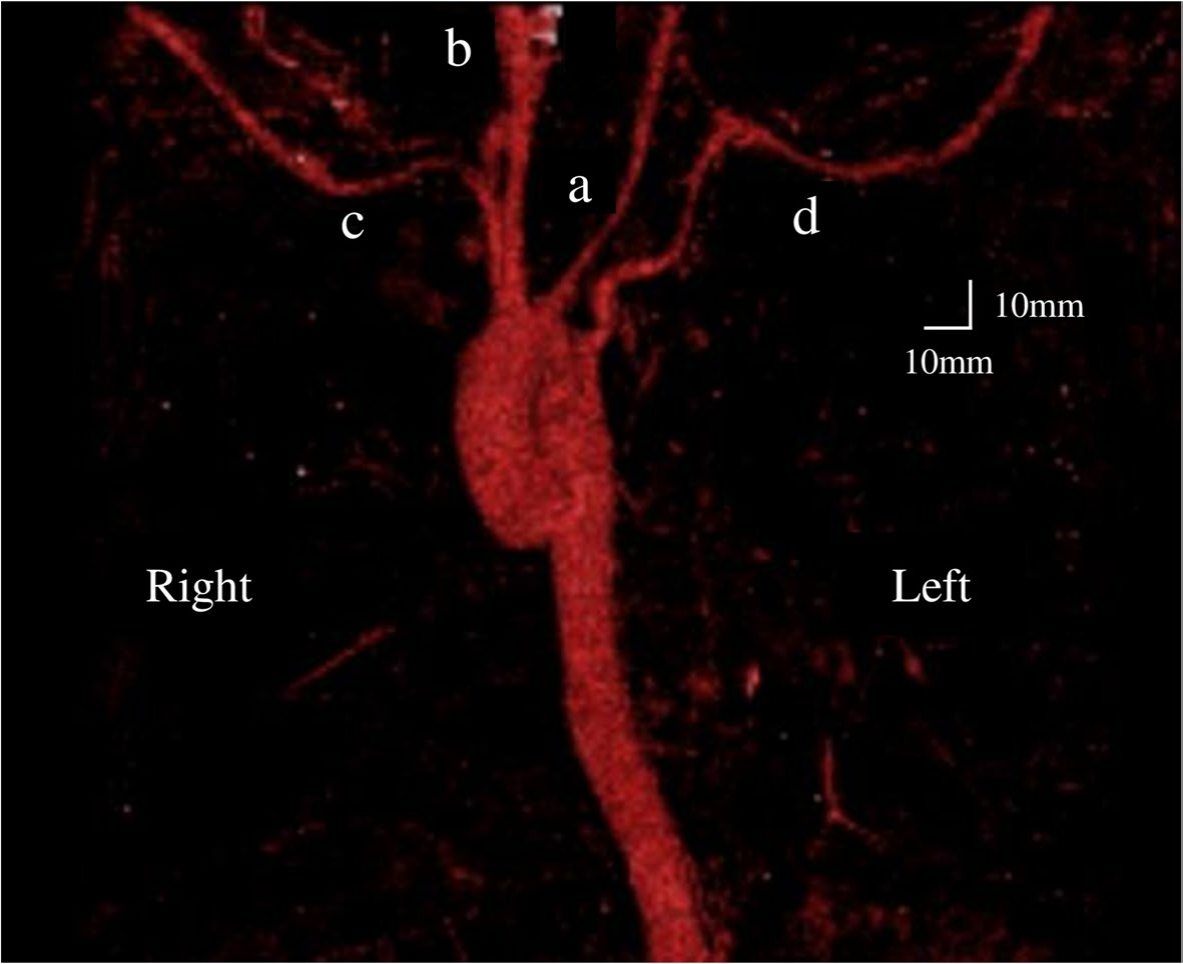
Three-dimensional computed tomography with vascular reconstruction. The left common carotid (a), right common carotid (b), right subclavian (c), and left subclavian arteries (d) branched sequentially from the proximal segment of the right-sided aortic arch. The left common carotid artery is narrower than the right common carotid artery. The left subclavian artery arises from the most distal portion of the right sided aortic arch and courses posterior to the esophagus, consistent with an aberrant left subclavian artery
